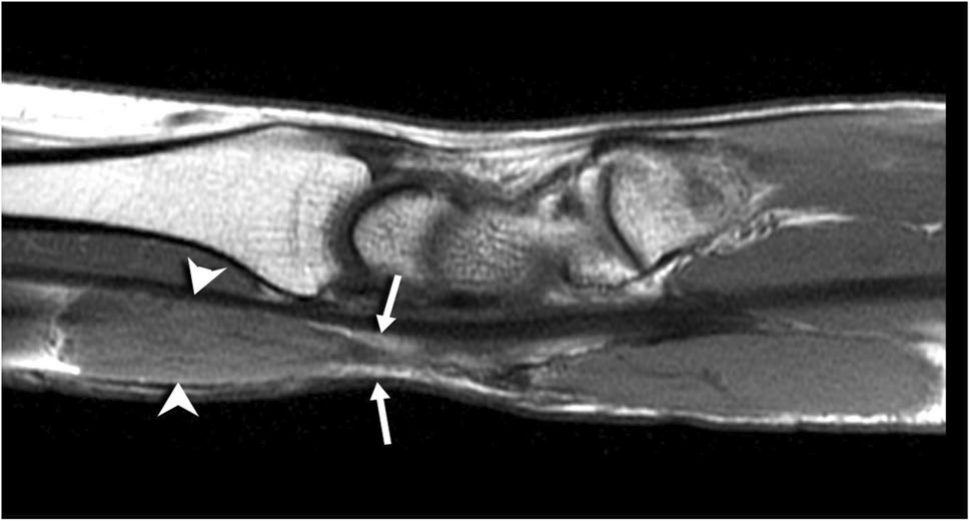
Sagittal T1-weighted fat-suppressed MR image shows the presence of a “split fat” sign and “tail sign,” with hyperintense adipose tissue (fat) surrounding the exiting median nerve (arrows) at the distal end of the mass. The mass itself is isointense to skeletal muscle (arrowheads)
